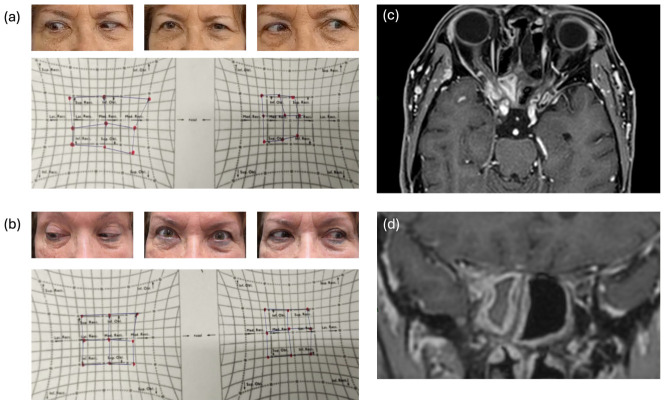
Case 4. (a) Gaze positions and Hess screen at presentation demonstrating right eye abduction deficit. (b) Post-treatment gaze positions and Hess screen showing near-complete recovery with minimal residual abduction limitation. (c,d) Contrast-enhanced, fat-suppressed T1-weighted MRI in axial and coronal planes demonstrating right sphenoidal sinusitis with extension to the orbital apex and cavernous sinus.
